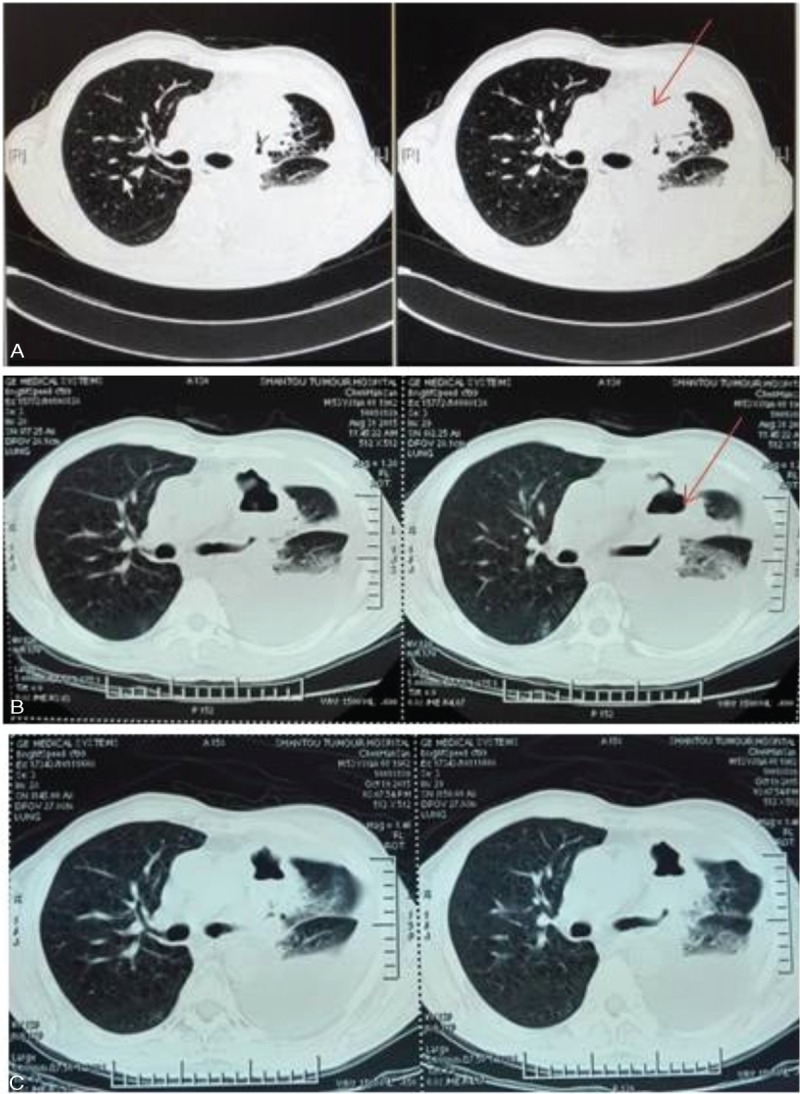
CT shows the mass in the lung (arrow indicate), (A) before apatinib treatment (July 2015). (B), (C) Apatinib treatment for 1 month and 3 months, respectively; local cavity can be seen. CT = computed tomography.
